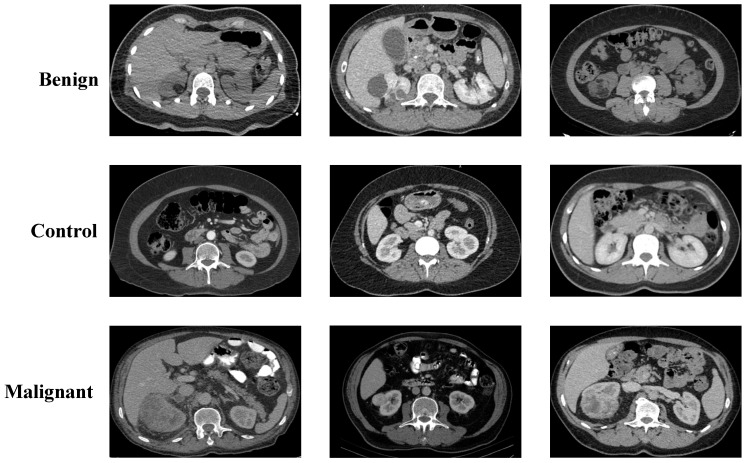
Sample axial CT images representing benign, control, and malignant kidney cases from the collected dataset.
